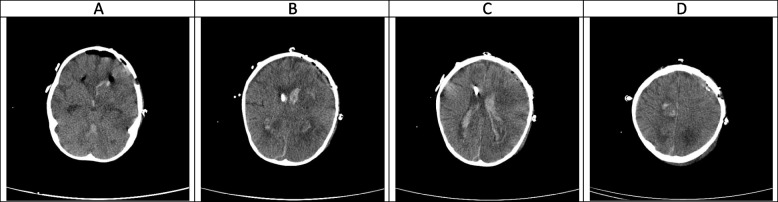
(the brain CT SCAN without contrast, day 3). A-C The degree of intracranial and intraparenchymal air,as well as the edema and post-operation changes of the left frontotemporal lobe decreased, however significant intraventricular hemorrhage is again seen, but with no sign of associated hydrocephalus, suggestive of the well-functioning EVD. D hemorrhagic lesion of high convexity of the right frontoparietal junction is partially improved
